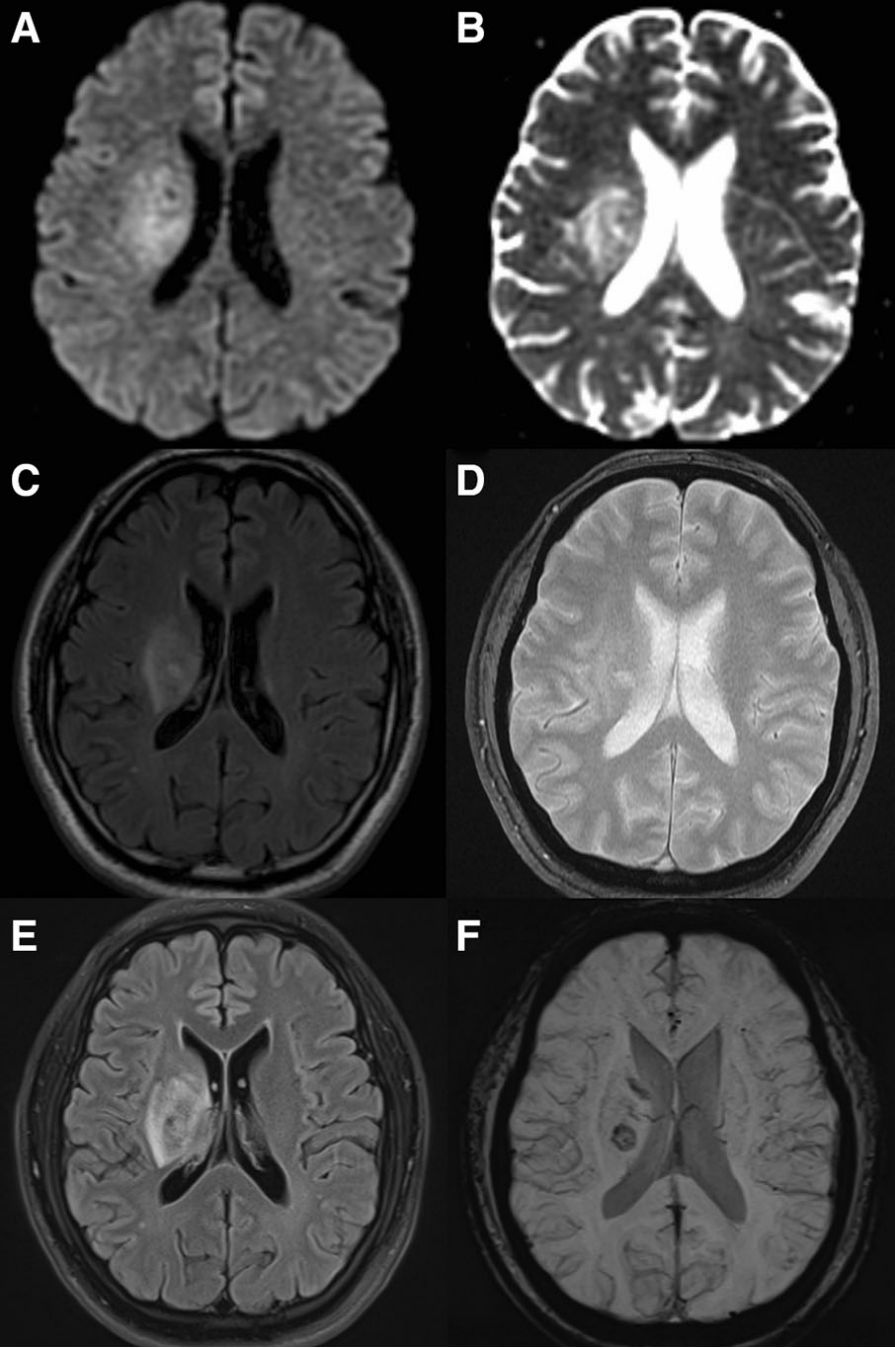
Initial and follow-up magnetic resonance images (MRI) at the first event. The initial MRI shows high signal intensity on diffusion-weighted image (DWI, a), without low signal intensity on apparent diffusion coefficient (ADC, b). Fluid-attenuated inversion recovery (FLAIR, c) images reveal increased signal intensities in right basal ganglia and centrum semiovale. No hemorrhagic lesion was detected in gradient-echo images (GRE, d). The follow-up MRI shows slightly increased extent of FLAIR high signal intensity area (e) and development of focal hemorrhage at right basal ganglia (f), compared to the initial MRI images
